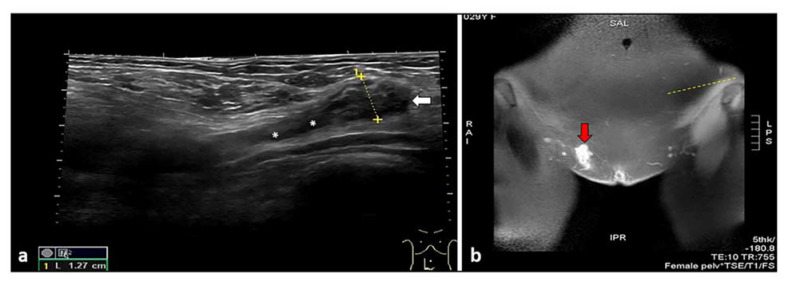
Extended field-of-view sonogram shows a hypoechoic and nodular mass (white arrow) located at the level of the Nuck canal (white asterisks) (a). Coronal T1-weighted MR image showing a hyperintense, pseudo-nodular mass (red arrow), confirming the presence of a hemorrhagic focus inside the inguinal canal representing endometriosis foci (b).
